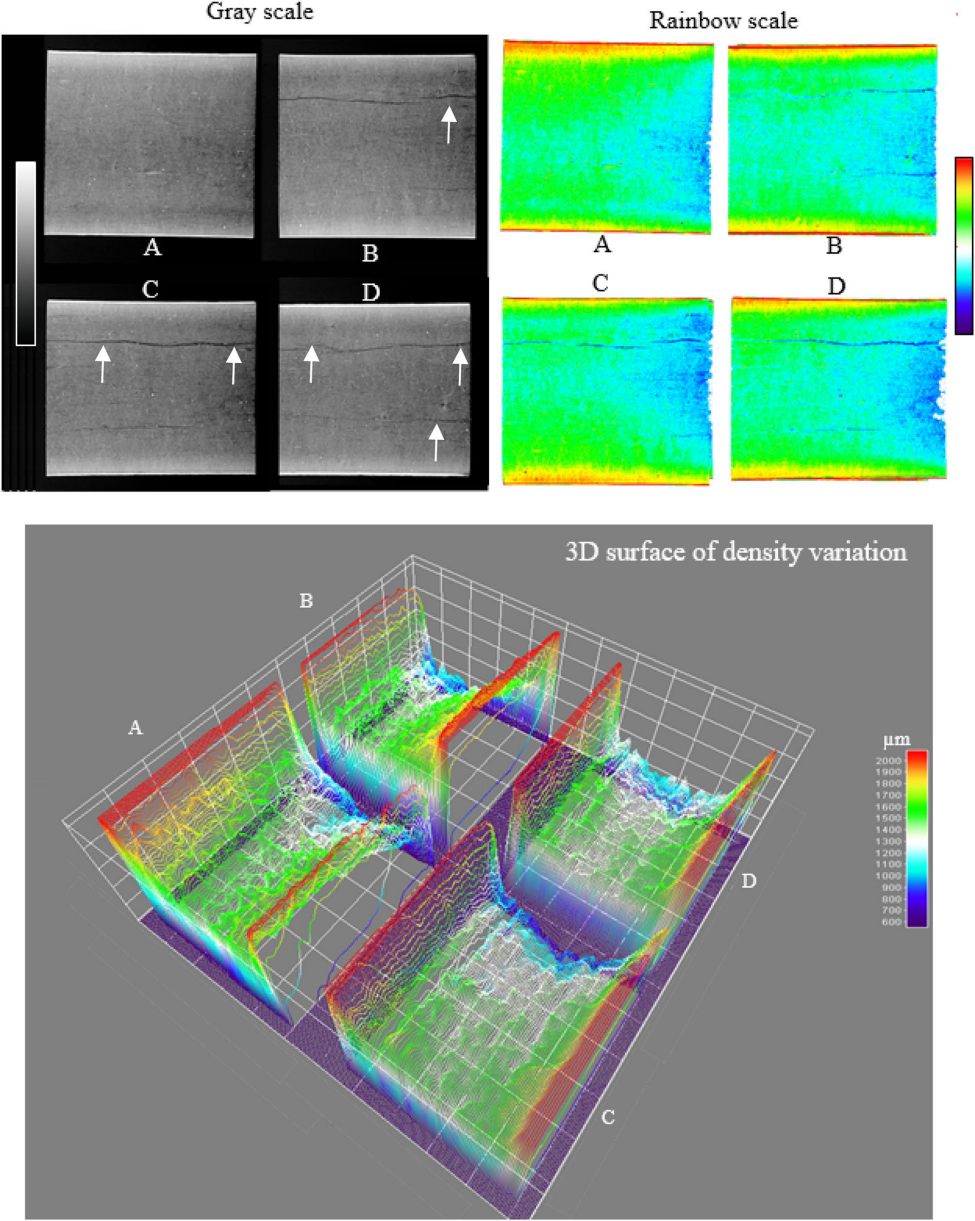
Digital X-ray images of MDF coated residues in gray and rainbow scales and 3D density variation plot. Untreated (A); torrefaction for 20 (B), 30 (C) and 40 (D) minutes. Areas with red and blue color indicate higher and lower density, respectively. White arrows indicate the cracks/fissures.
